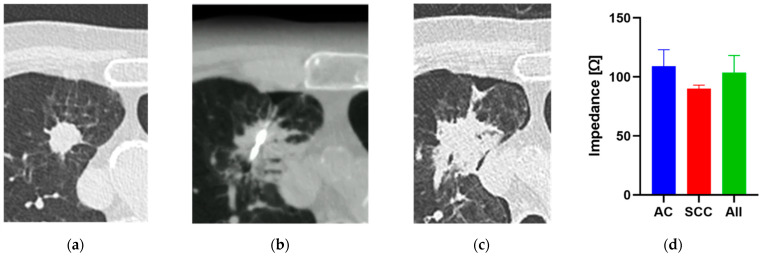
Example of a radio-frequency ablation (RFA) performed percutaneously using a bipolar probe. Displayed are (a) CT images before insertion of the RFA probe into the lung tumor mass, (b) the formation of necrotic tissue during RFA, (c) the formation of necrotic tissue after RFA, and (d) the recorded electrical impedance of the tumor (type: adenocarcinoma (AC) and squamous cell carcinoma (SCC)) throughout the procedure.
